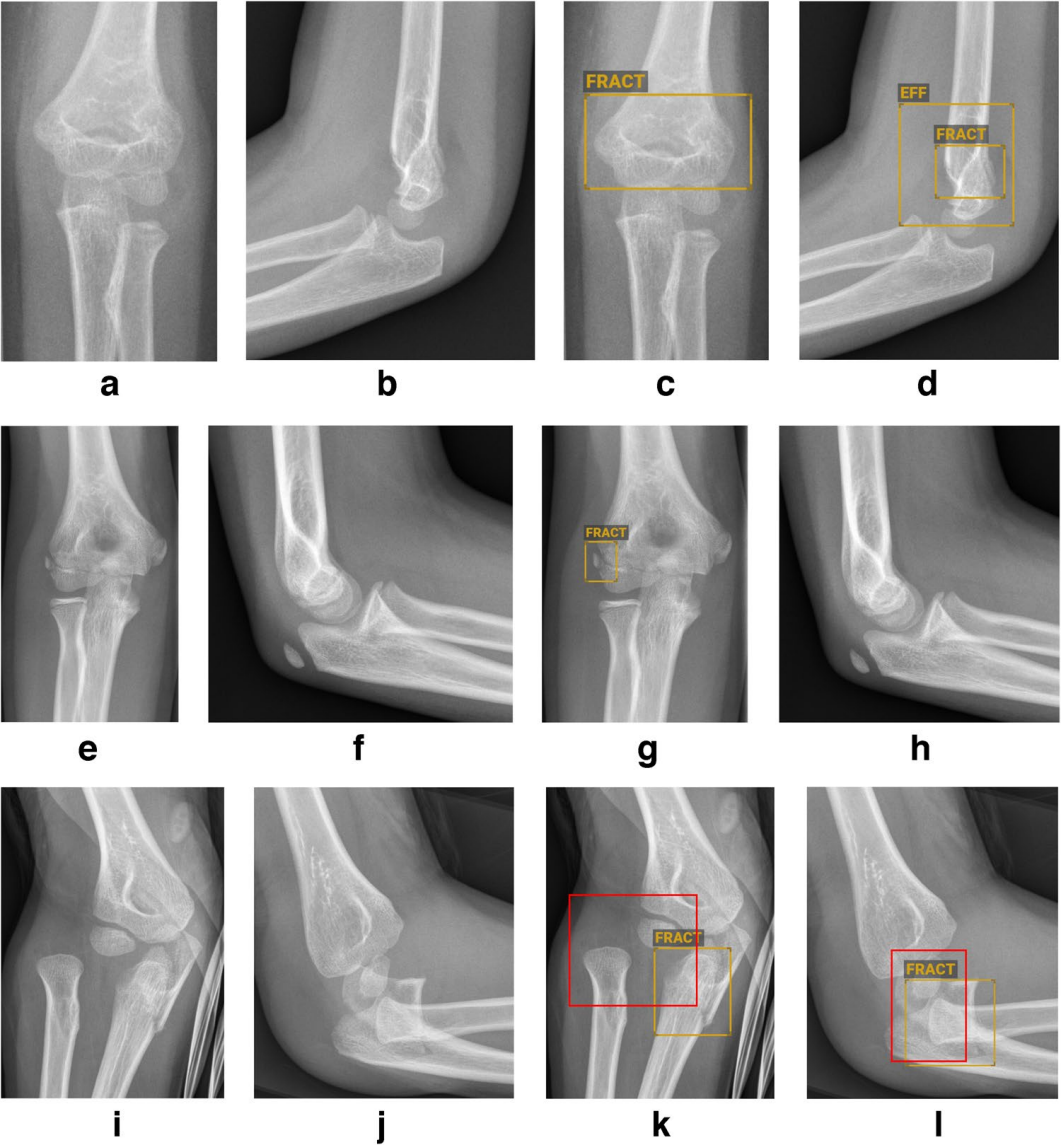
Examples of elbow radiographs showing true positive (a–d), false positive (e–h) and false negative (i–k) diagnoses made by BoneView (Gleamer, Paris, France). a–d Anteroposterior (AP) and lateral radiographs of the left elbow in a 4-year-old girl before (a,b) and after (c,d) annotation by BoneView. There is a complete supracondylar fracture with effusion (positive anterior and posterior fat pad sign). The fracture was detected with a confidence score above 90% (*rectangles/“FRACT”*). The effusion was depicted correctly by BoneView (*rectangle/“EFF”*). e–h AP and lateral radiographs of the right elbow in a 10-year-old girl before (e,f) and after (g,h) annotation by BoneView. The radiographs were normal. BoneView detected an avulsion of the radial epicondyle with a confidence score above 90% (*rectangle/“FRACT”*). i–l AP and lateral radiographs of the right elbow in a 6-year-old boy before (i,j) and after (k) annotation by BoneView. There is a Monteggia fracture-dislocation. BoneView detected the true positive complete fracture of the olecranon with a confidence score above 90% (*orange rectangle/“FRACT”*). The associated proximal radial dislocation was disregarded (*red rectangle*)
